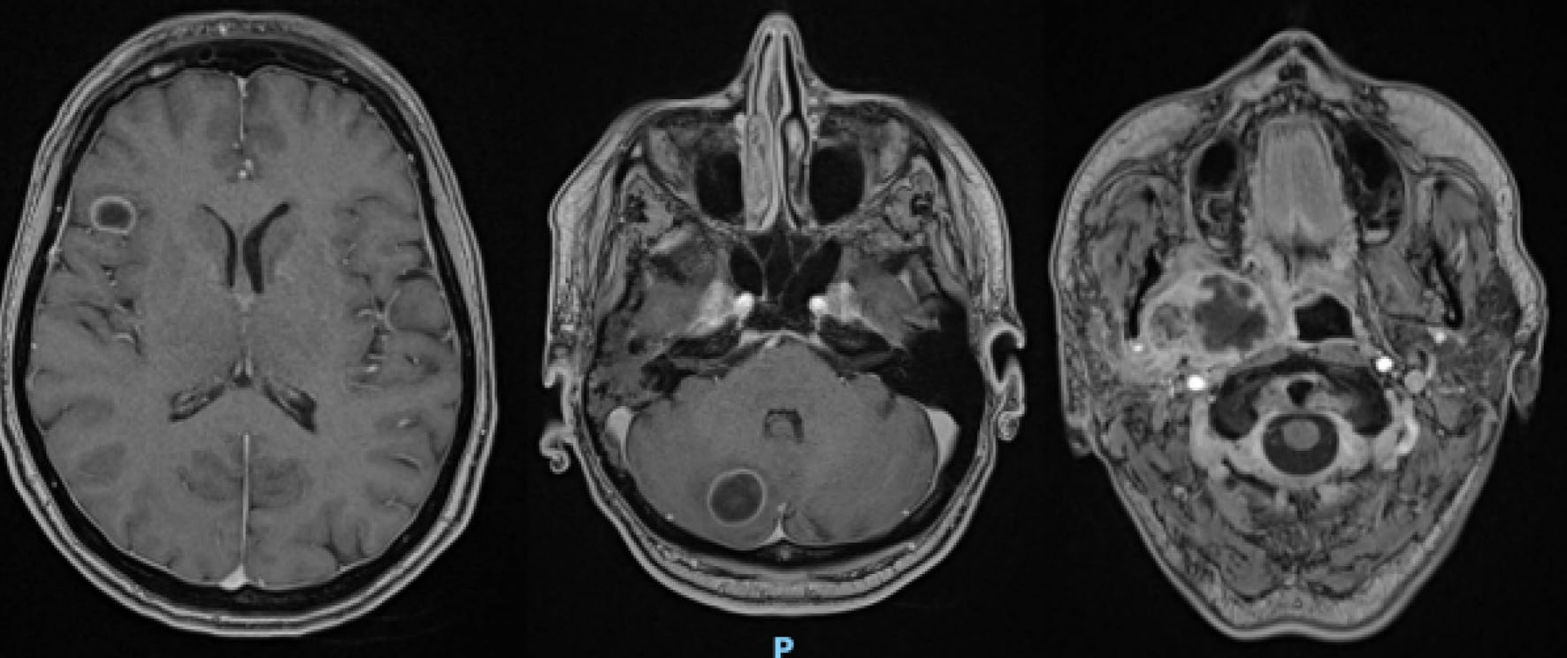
Brain MRI with transverse scans (T1_vibe_dixon sequencies). The presence of a pathological formation in the right lateral pharynx with a mass effect on the airways, with a non-vascularized internal portion, was confirmed; the two lesions at the encephalic and cerebellar level were confirmed, with the diagnosis of neoplastic lesions
